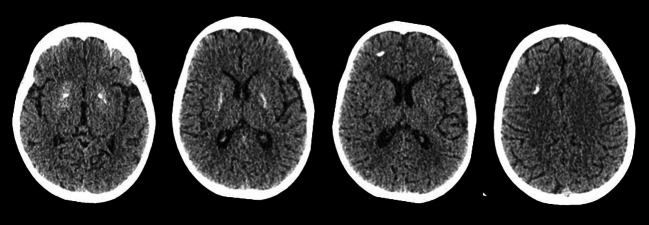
A cranial CT in a patient with biallelic *ACP5* mutations. Imaging in Patient 21 at nine years of age demonstrated intracranial calcification of the white and grey matter junction and bilateral blush and spots in the head of the caudate, putamen and globus pallidus and deep gyral matter on the right and left
